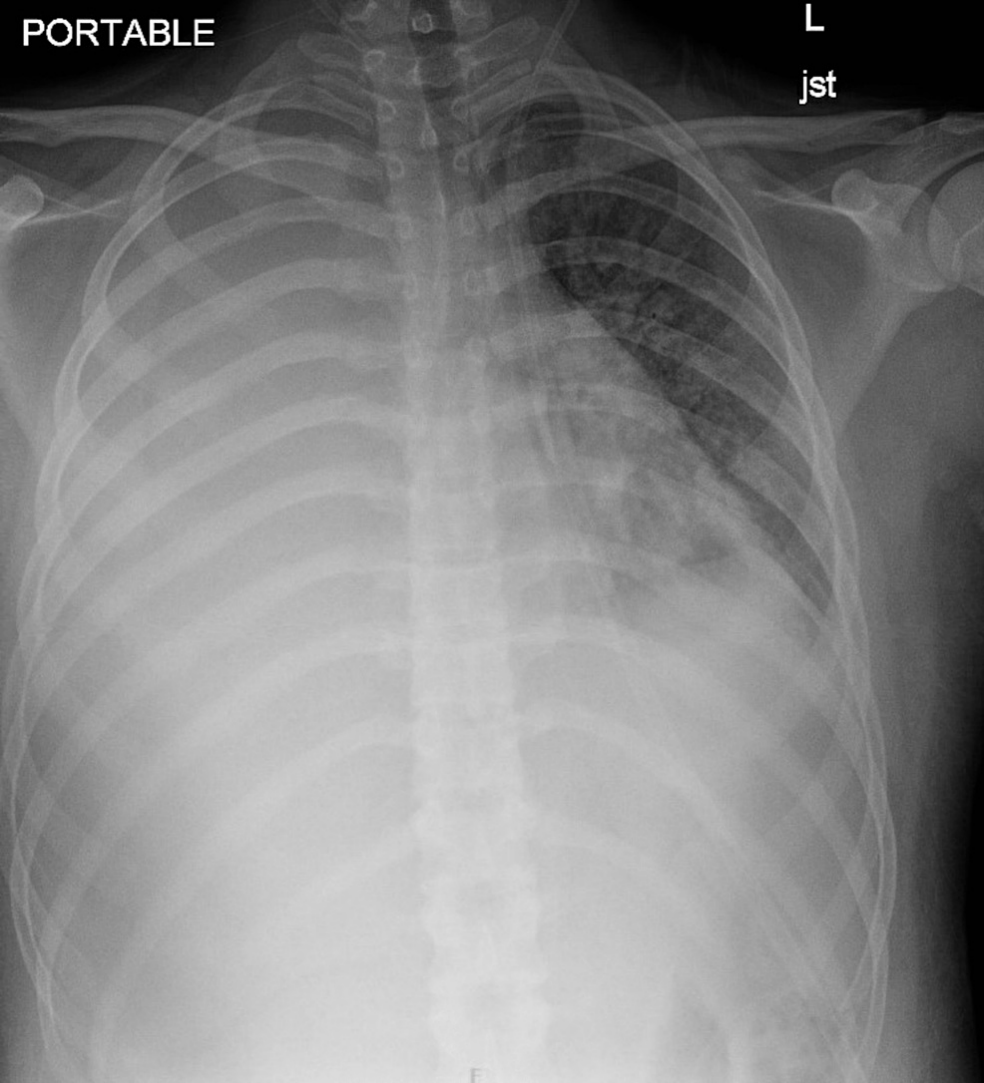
Chest X-ray on day five of hospitalization Complete opacification of the right lung and large right pleural fluid was observed. There were new interstitial and patchy airspace opacities in the left perihilar region and lung base.
